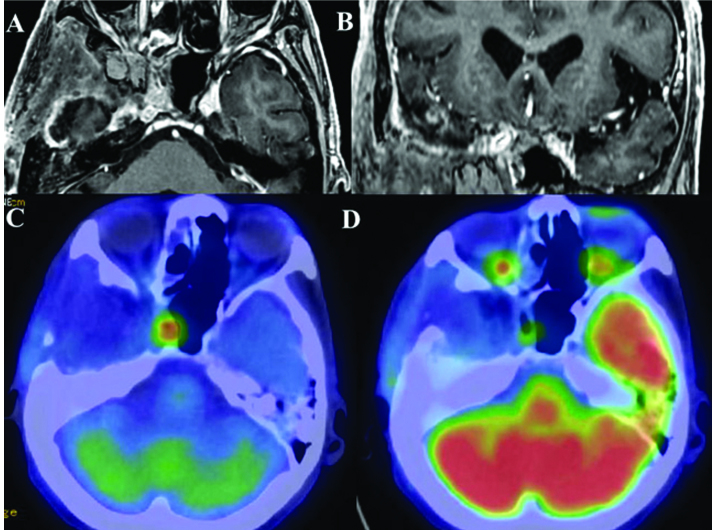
Post-operative examination. (A, B) Enhanced MRI and (D) FDG-PET were unable to visualize the remnant tissue. (C) ^11^C-methionine-PET clearly revealed the remnant tissue of the ameloblastic carcinoma around the right internal carotid artery.
